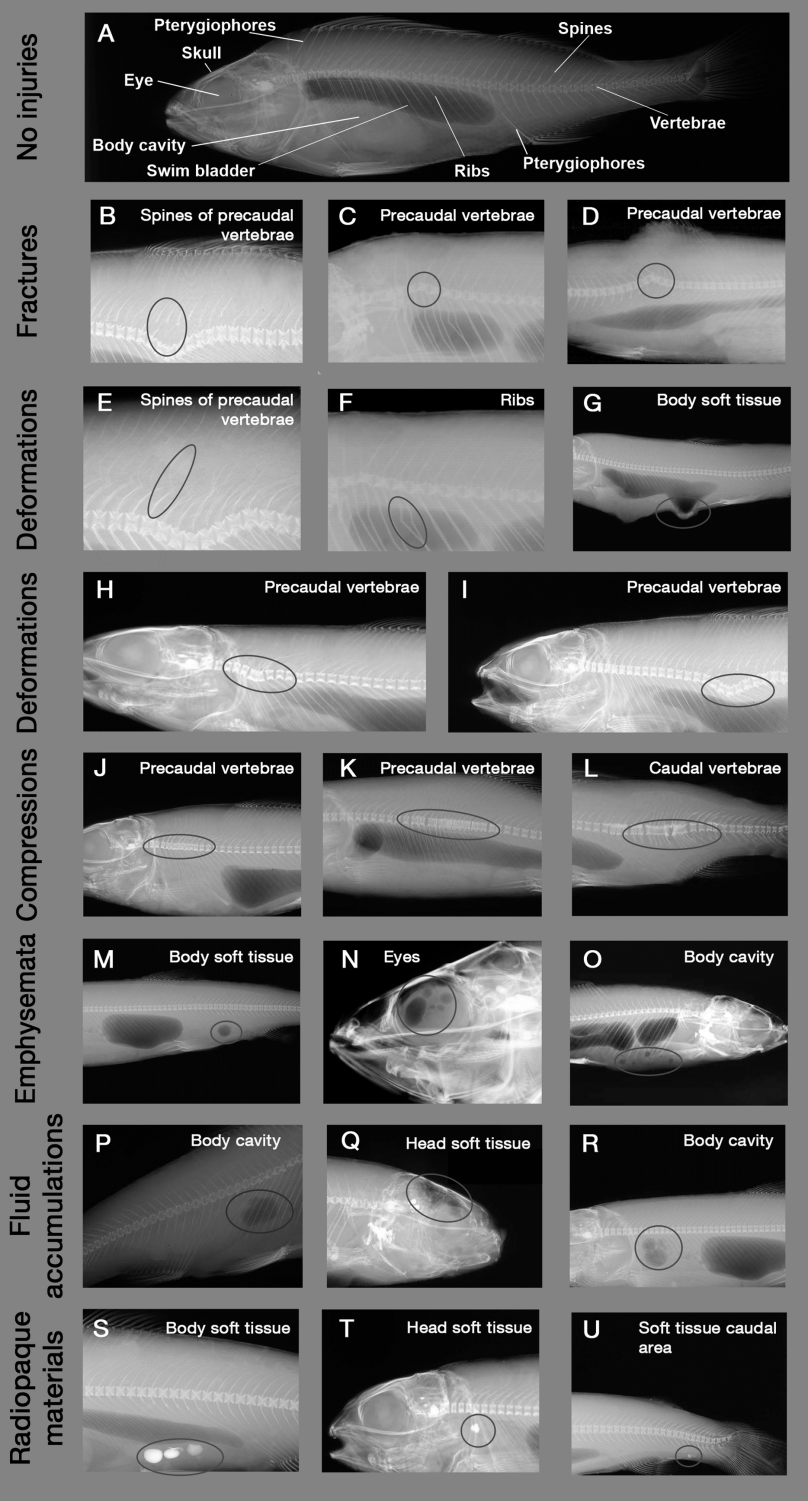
Example X-ray images of skeletal and soft tissue injuries. Encircled body parts indicate injured areas. (A) Reference fish with no injuries (*Perca fluviatilis*), (B–D) Fractures, (E–G) Deformations of spines, ribs and soft tissue, (H and I) Deformations of vertebrae, (J–L) Compressions, (M–O) Emboli, (P–R) Fluid accumulations, (S–U) Radiopaque materials. Source: Institute of Anatomy, Faculty of Medicine, LMU Munich, Munich, Bavaria, Germany.
